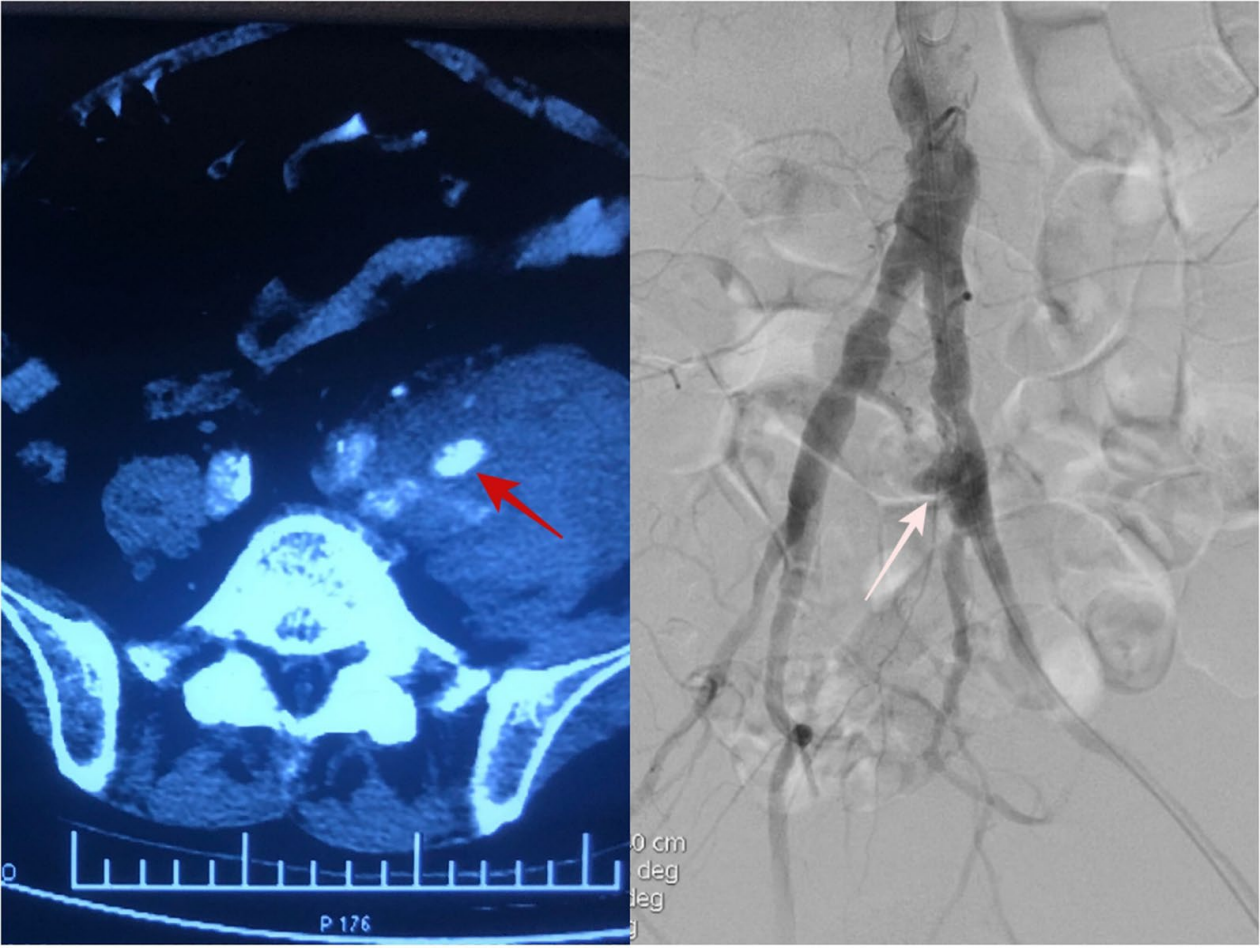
Red arrow: enhanced CT showed hematoma around the left iliac artery and contrast medium extravasation. White arrow: the angiography of the abdominal aorta showed localized outward bulging of the left lower common iliac artery and sign of contrast medium extravasation
